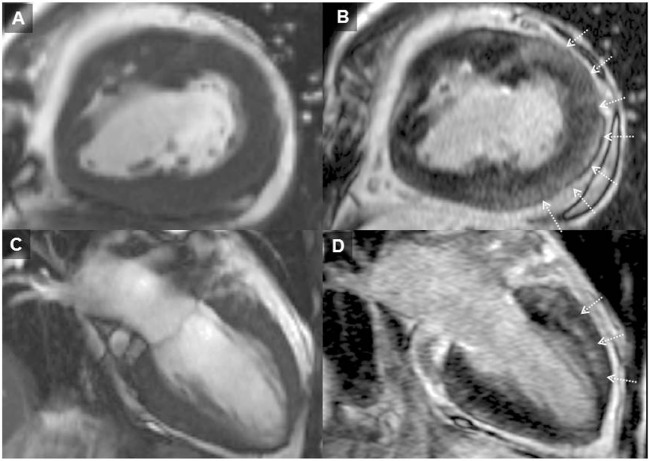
Cardiovascular magnetic resonance images status post-lateral tunnel total cavopulmonary connection surgery for single-left ventricle (LV) physiology. (A) and (C) (2D cine images) compared to (B) and (D) (LGE images). Arrows represent diffuse appearance late gadolinium enhancement in the free wall of the LV, which is the primary ventricle. This kind of fibrosis pattern in Fontan is one of the several previously described patterns (82).
